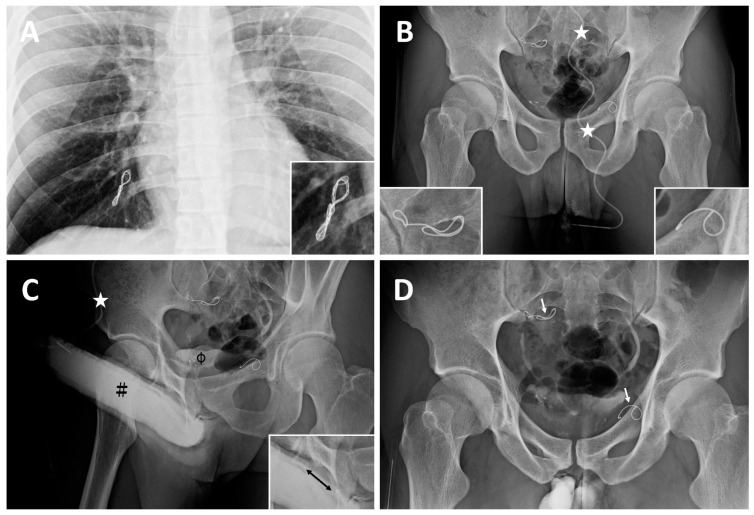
Imaging of a banker born in 1985. (A) In August 2016, a chest X-ray disclosed a dumbbell-shaped twisted coil complex lodged in the right pulmonary arterial avenues (magnified insert; bottom right). It was inserted in 2008 for the first CE. (B) A 19G scalp needle (white asterisk) was fixed while the needle tip was inserted intracavrnously. A KUB showed two coils lodged at the bifurcation level of the right iliac vein (magnified in the insert; bottom right) and left internal pudendal vein (magnified in the insert; bottom left), which corresponded to the coils inserted in 2010 and 2012, respectively. Those two coils were noted to be outside the venous channels as they were not in the venous vessel course but perpendicular to it. (C) Although artificial erection was induced via PGE-1 vasoactive agent, its rigidity was undermined by erection-related veins (black double-head arrow; bottom left insert) mounting the dorsal groove concave of the corpora cavernosa (black pump) and urinary tract (black hash). (D) After penile venous stripping, intracorporeal retention was pronounced at the penile crura and the coils remained stationary. Note a tapeworm-shaped coil was noted in the region of the right iliac vein (white arrow) and a pigtail-shaped coil was shown at the left internal pudendal vein level (white arrow), they are at 90 degrees to the venous channel.
